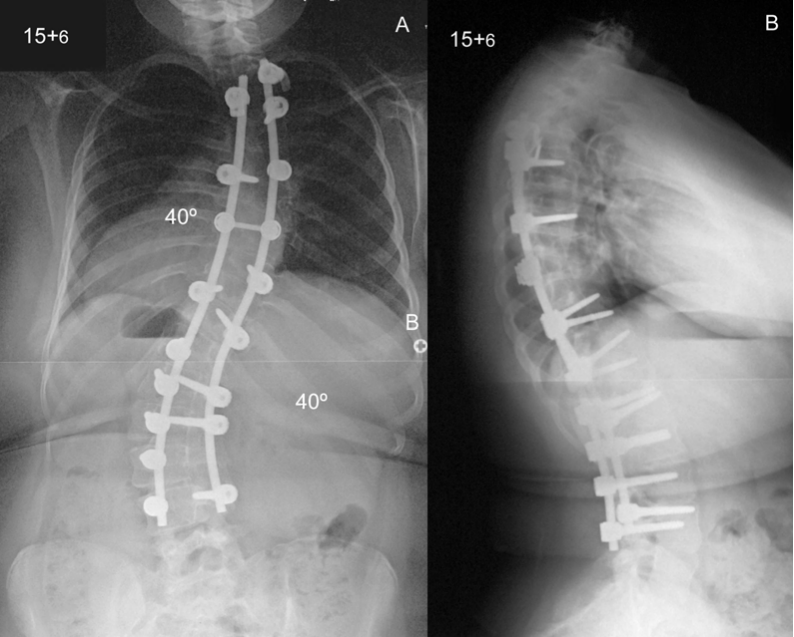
Postoperative posteroanterior (A) and lateral (B) radiographs of the spine show correction of the thoracic and lumbar scoliosis to 45 and 40° and a balanced spine in both the coronal and sagittal planes.
